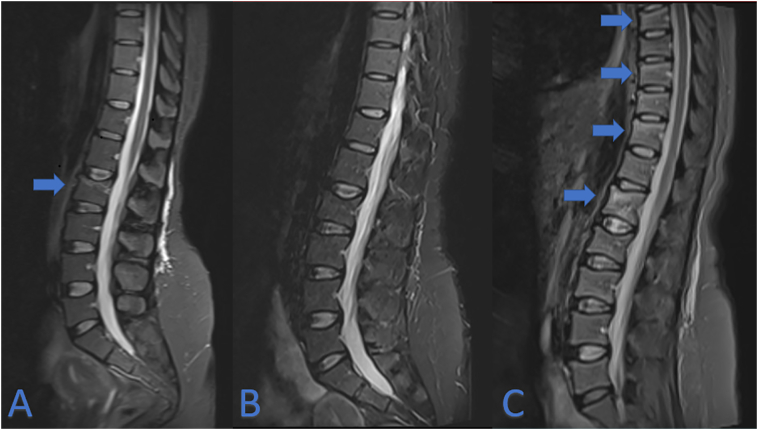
MRI T2-images of the lumbar spine region (A) April 2014, (B) May 2019, (C) February 2021. Blue arrows indicate bone oedema compatible with recent fractures. In A: L2. In C: L1, Th7, Th9 and Th11.
